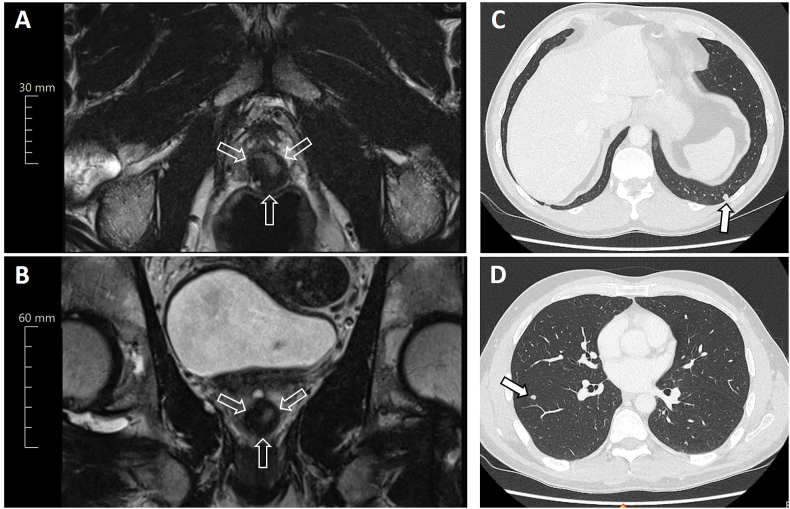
Pelvic magnetic resonance imaging shows a 2 cm prostatic mass on (A) axial and (B) sagittal T2-weighted images as indicated by open arrows. (C and D) Subsequent chest CT imaging reveals multiple suspicious bipulmonary nodules (maximum diameter 8 mm; indicated by filled arrows).
